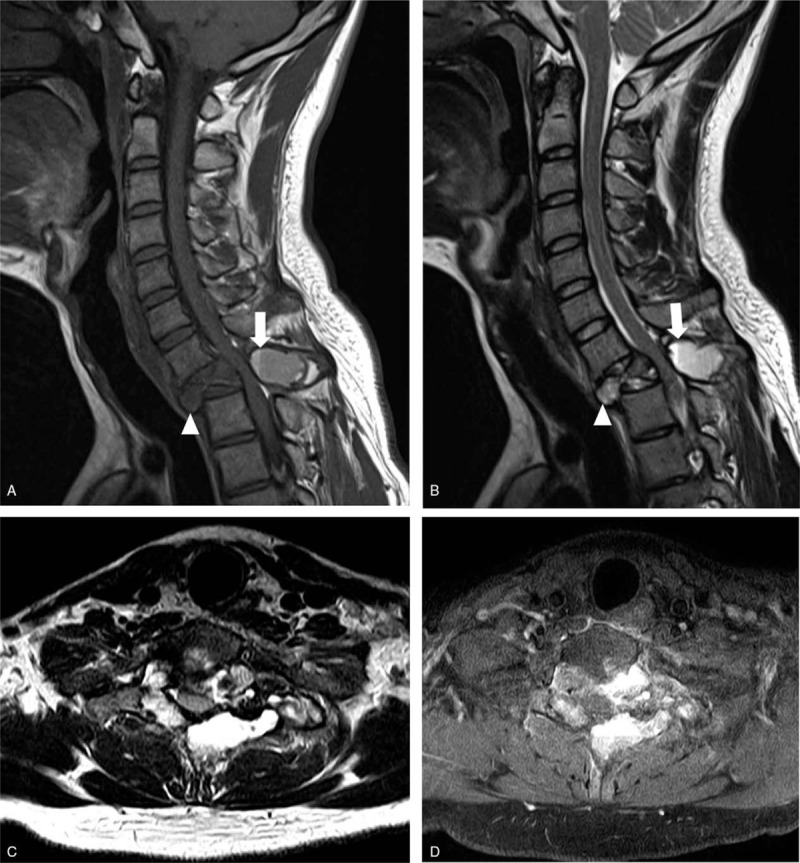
Preoperative magnetic resonance imaging. (A) and (B) T1- and T2-weighted sagittal image reveled a bony cystic mass with internal septation (arrow head) and fluid-fluid levels (arrow) at the T1 vertebra. (C) T2-weighted axial image showed multiple cysts and internal septations, and these cyst walls and internal septations were enhanced on T1-weighted image (D).
